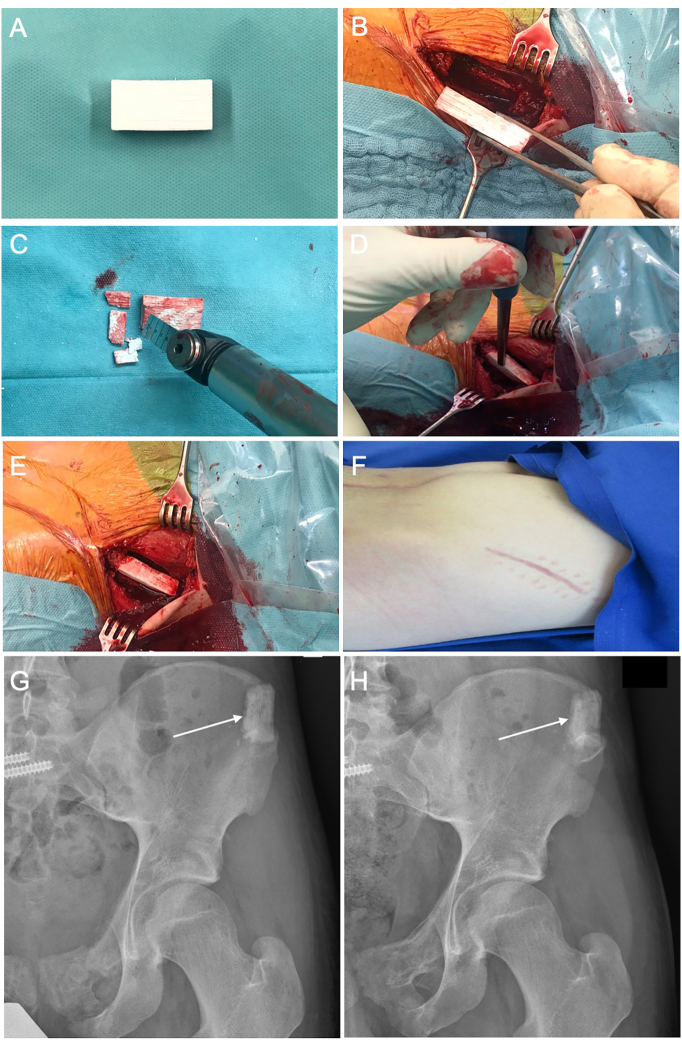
Block shaped scaffold of rattan-wood based not-sintered hydroxyapatite and beta-tricalcium phosphate material (b.Bone™, GreenBone ORTHO S.p.A Faenza, Italy) with a size of 40x30x10 mm (A). Defect at the left iliac crest after tricortical bone graft harvesting with a defect size of 30 × 20 × 10 mm. Customizing of the biomaterial with oscillating saw for press-fit implantation by shortening of the block by 9 mm (C) and press-fit insertion of the scaffold into the defect with a pestle (D). Press-fitted implant into the iliac crest (E). Uneventful wound healing without excessive scar formation after 12 weeks over the left iliac wing (F). Post-operative X-ray showing complete filling of the iliac crest defect after tricortical bone graft harvesting with correct placement of the scaffold biomaterial (G) with clearly visible bone-implant interface (arrow). Post-operative X-ray after 8 months revealing fragmentation and good bony integration of the scaffold with bone bridging of the bone-implant interface region (arrow) from the surrounding host bone into the implant (H).
